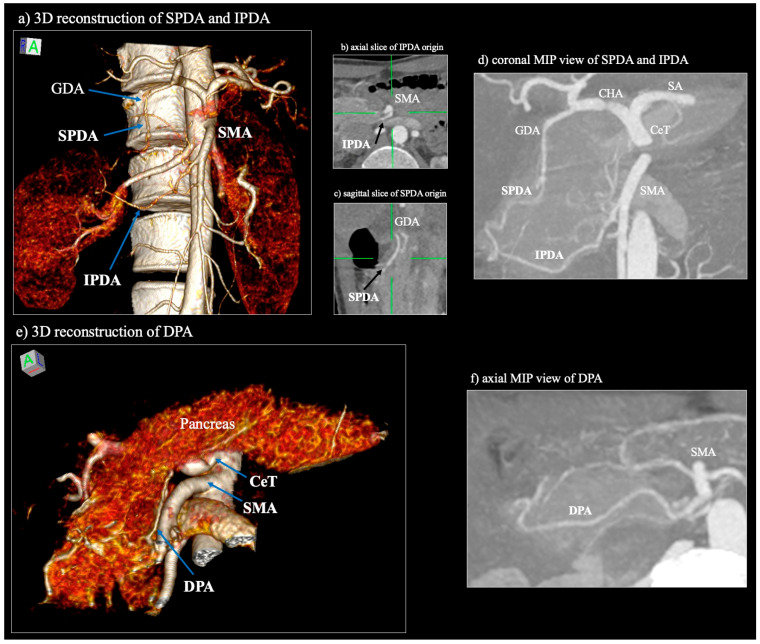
Example of the computed tomography angiography of a 42-year-old female patient depicting the main pancreatic arteries. CeT—coeliac trunk; SMA—superior mesenteric artery; GDA—gastroduodenal artery; CHA—common hepatic artery; SPDA—superior pancreaticoduodenal artery; IPDA—inferior pancreaticoduodenal artery; DPA—dorsal pancreatic artery; SA—splenic artery.
